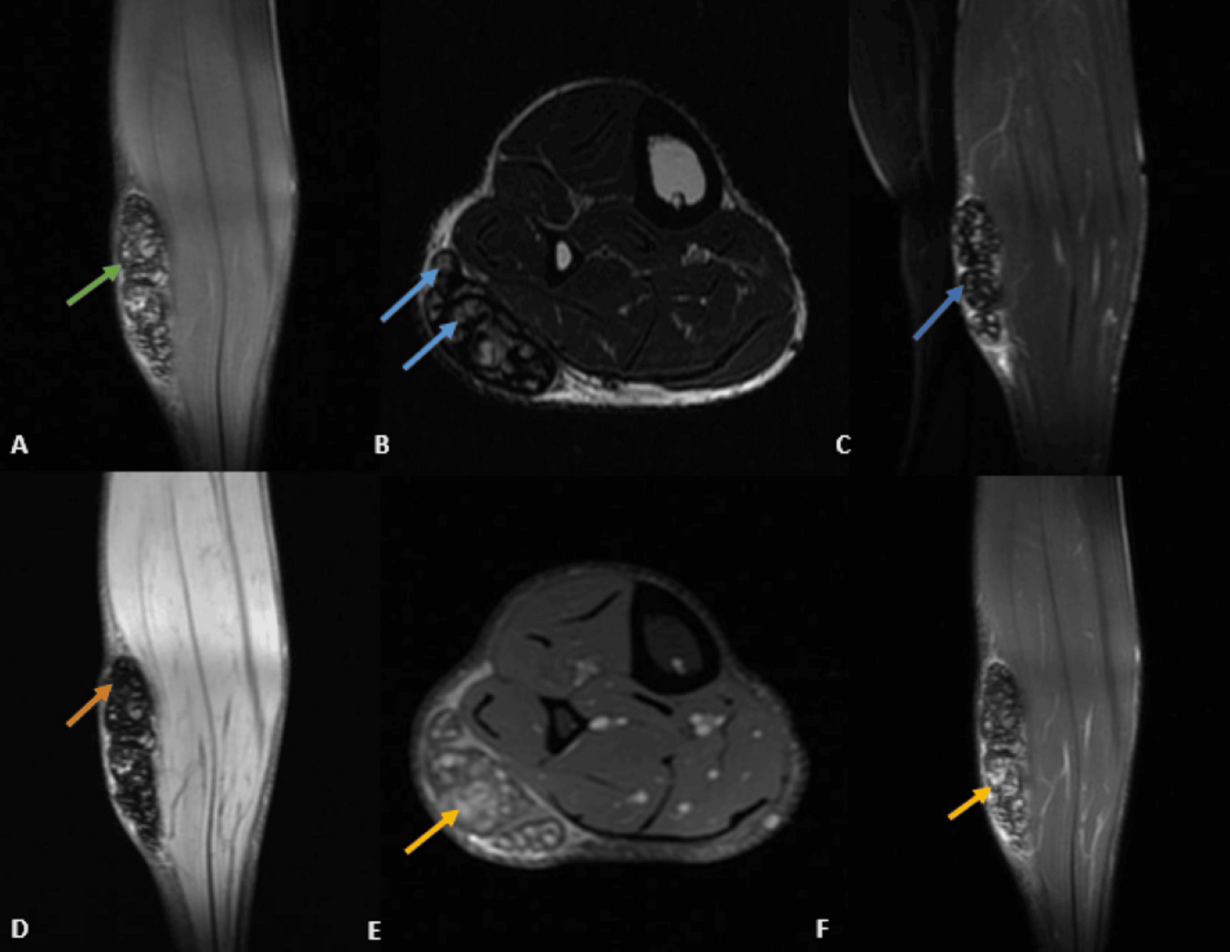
On further MRI, a large, well-defined, ovoid, lobulated, heterogeneous altered signal intensity lesion measuring 3.9 x 2.2 x 9.8 cm was noted in the subcutaneous plane of the postero-lateral aspect of the right leg, overlying the fibularis longus and soleus muscles (mid-shaft region). (A) On T1 (coronal), the lesion (green arrow) was isotense to hypointense compared with the skeletal muscles. (B, C) On T2 (axial) and STIR (coronal), it appeared heterogeneous with multiple internal hypointense septa/reticulations. Few areas of the dot in the circle were observed (blue arrows). (D) On MERGE (coronal), ovoid areas of blooming are noted within (orange arrow). Mild edema of the adjacent subcutaneous tissue was noted. (E, F) On post-contrast images (axial and coronal), it shows heterogeneous enhancement (yellow arrows). No evidence of any underlying muscular extension, abscess, or discharging sinuses was observed. MERGE, multiple echo recombined gradient echo; STIR, short tau inversion recovery.
